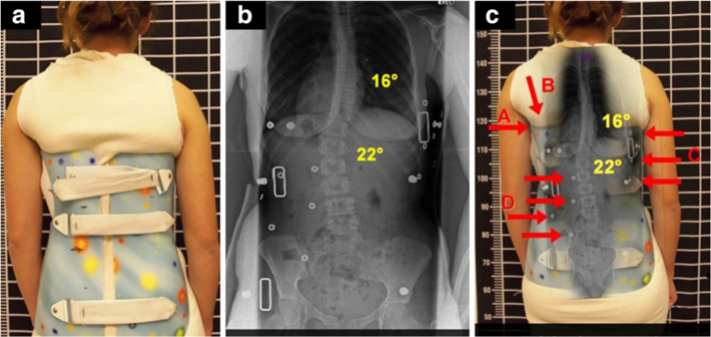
TLSO-type brace with low axilla, thoracic and lumbar pads. a: Patient (Nov 2013) in a TLSO-type brace which the patient reported to be a more or less comfortably fitting brace. b and c: In-brace X-ray of patient in TLSO type brace at one month presented right thoracic curve with the apex at T8 and left lumbar curve with the apex at L2. The thoracic pad was located at T10 to L1 and the lumbar pad was at L1 to L5 which could be considered theoretically correct. However in practical terms, these were too low when considering that only 2 cm to 3 cm of growth would cause these curves to go into progression. The in-brace X-ray presents a reduction of the curves from pre-brace of 22 degrees Cobb to in-brace 16 degrees Cobb and lumbar pre-brace of 27 degrees Cobb to in- brace of 22 degrees Cobb. The author fabricated a Cheneau-Rigo handmade type brace which had in brace correction from pre-brace thoracic 22 degrees Cobb to thoracic 3 degrees Cobb and pre-brace lumbar 27 degrees Cobb to 19 degrees in-brace Cobb.
